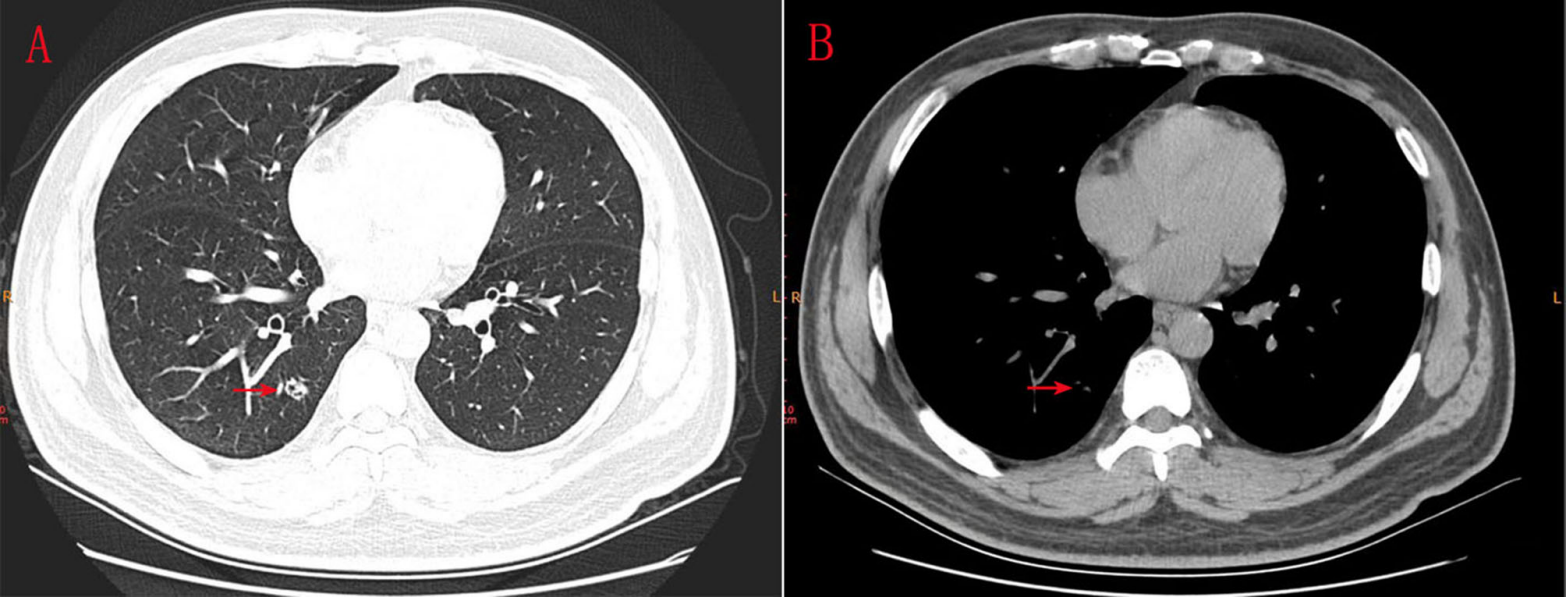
(A, B) CT scanning indicated an 9.7*1.1 millimeters mGGO in the posterior basal segment of inferior lobe of right lung.
